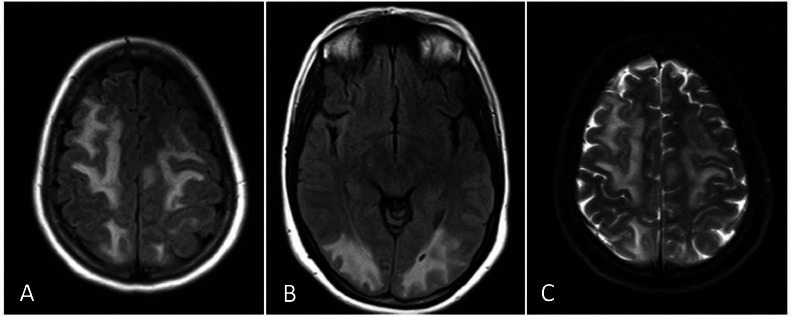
(A and B) Axial FLAIR (fluid-attenuated inversion recovery) and (C) Axial T2-weighted images show asymmetrical cortical, subcortical, and deep white matter with high signal intensity in fronto-parieto-occipital lobes.
